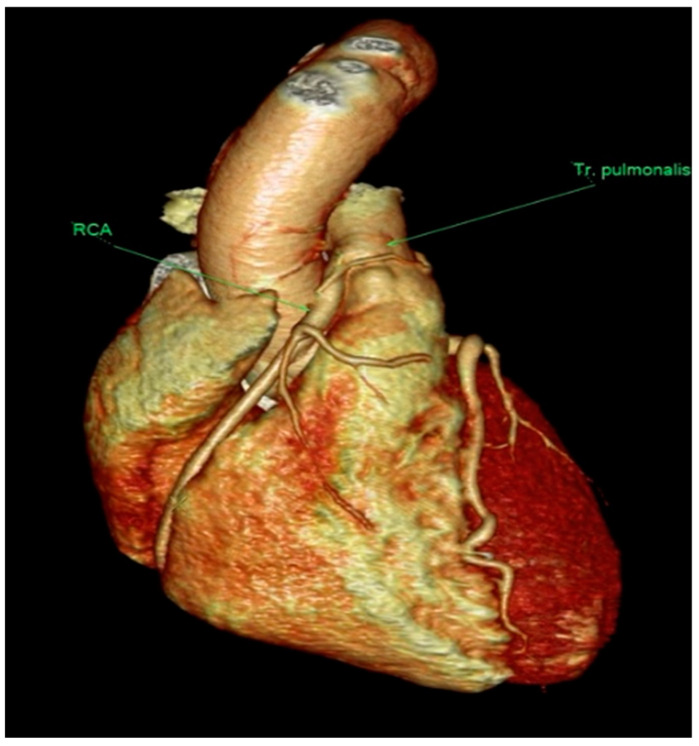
CT scan verification of the presence of ARCAPA syndrome.
